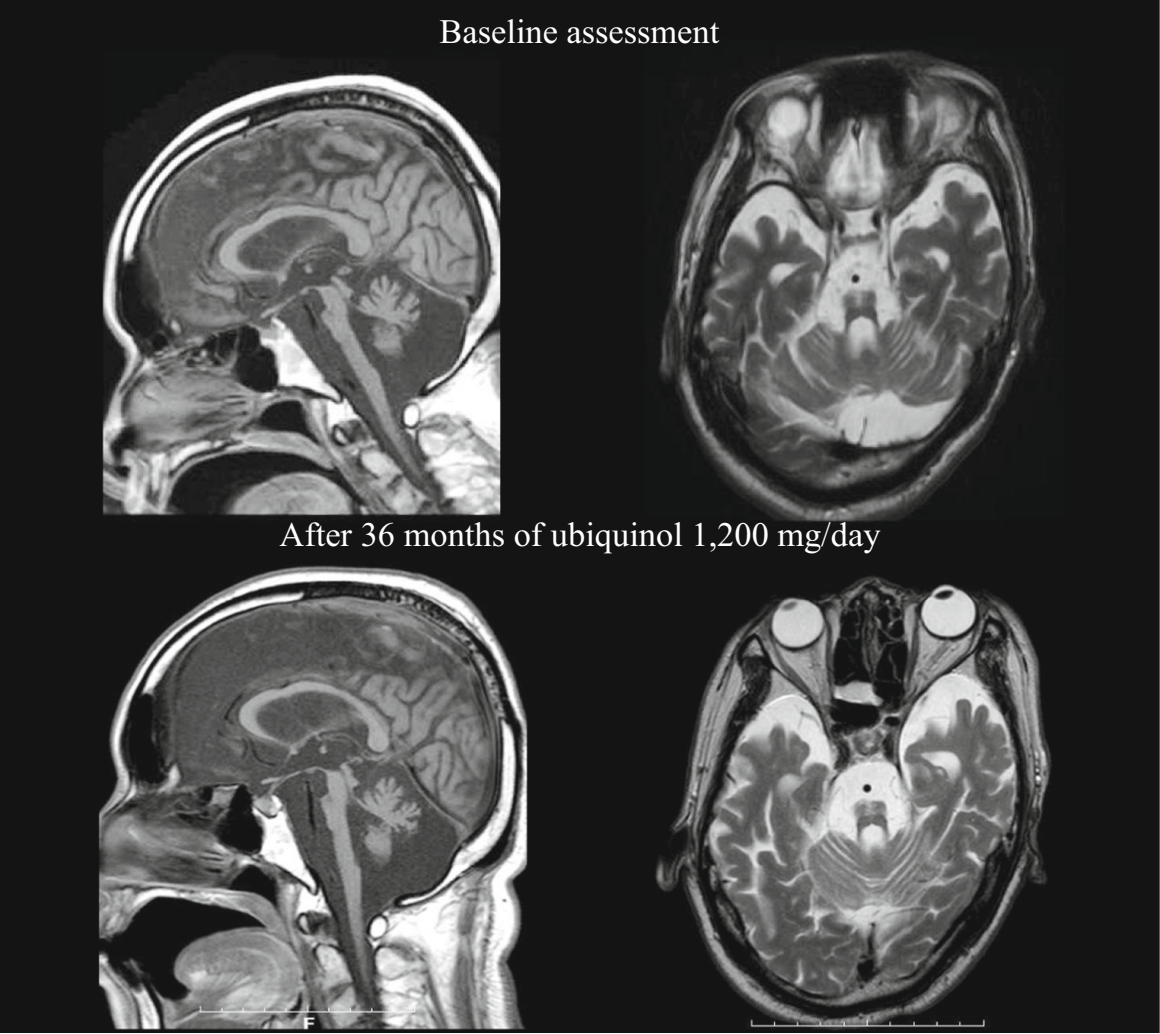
Brain MR images of patient. Upper images were obtained at 60 years of age (before supplementation of ubiquinol), and lower images were obtained at 63 years of age (after 36 months of ubiquinol 1200 mg/day). T1-weighted sagittal images (*left*) and T2-weightd axial images at the middle regions of the pontine base (*right*). They show gross atrophy of the infratentorial structures especially in the pons, middle cerebellar peduncle, and cerebellum
